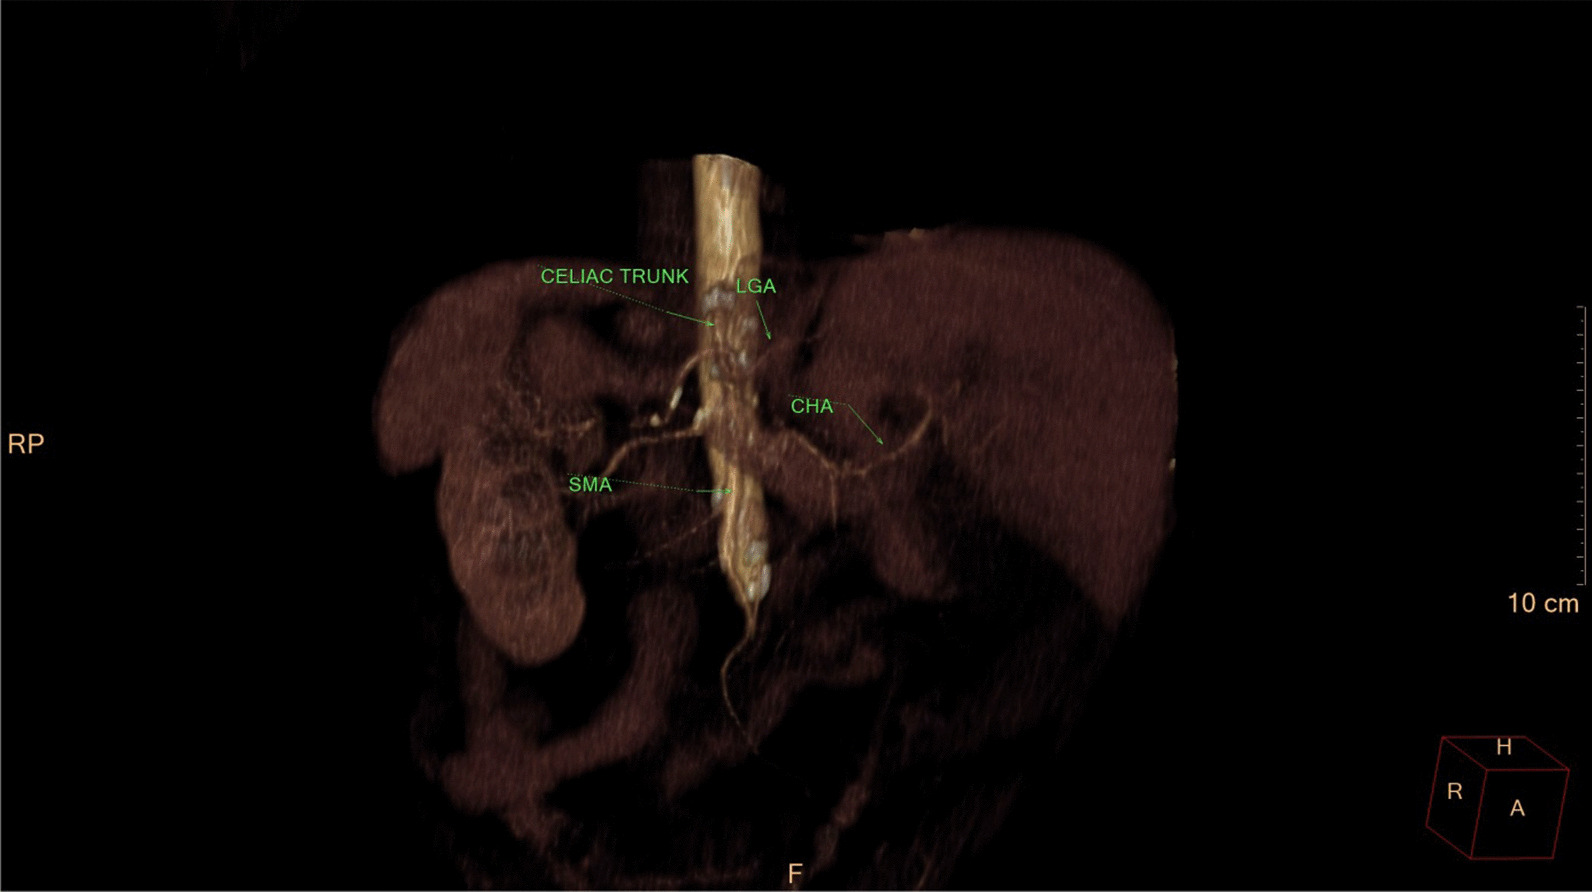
CT volume rendered 3D reconstruction. Variant anatomy of hepatic artery: replaced CHA, originating from the SMA and there is an accessory LHA from LGA
